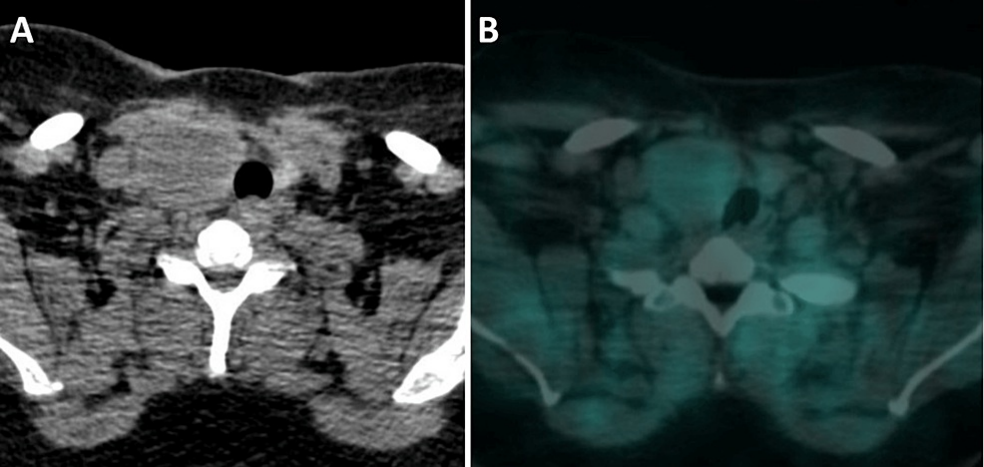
The 3-hour delayed (A) unfused axial CT and (B) fused axial SPECT/CT images from the first parathyroid scintigraphy reveal demonstrates asymmetrical enlarged right thyroid lobe. No abnormal tracer retention suggestive of a parathyroid adenoma.
